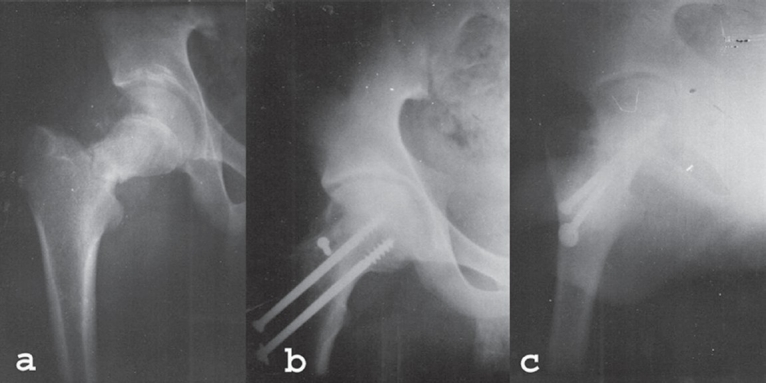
a,b,c: X-ray anteroposterior view of right hip shows femoral neck fracture (a), Two years followup X-rays AP and lateral views shows sound bony union and implant *in situ,* screw, used to fix muscle pedicle is visible (b and c)
